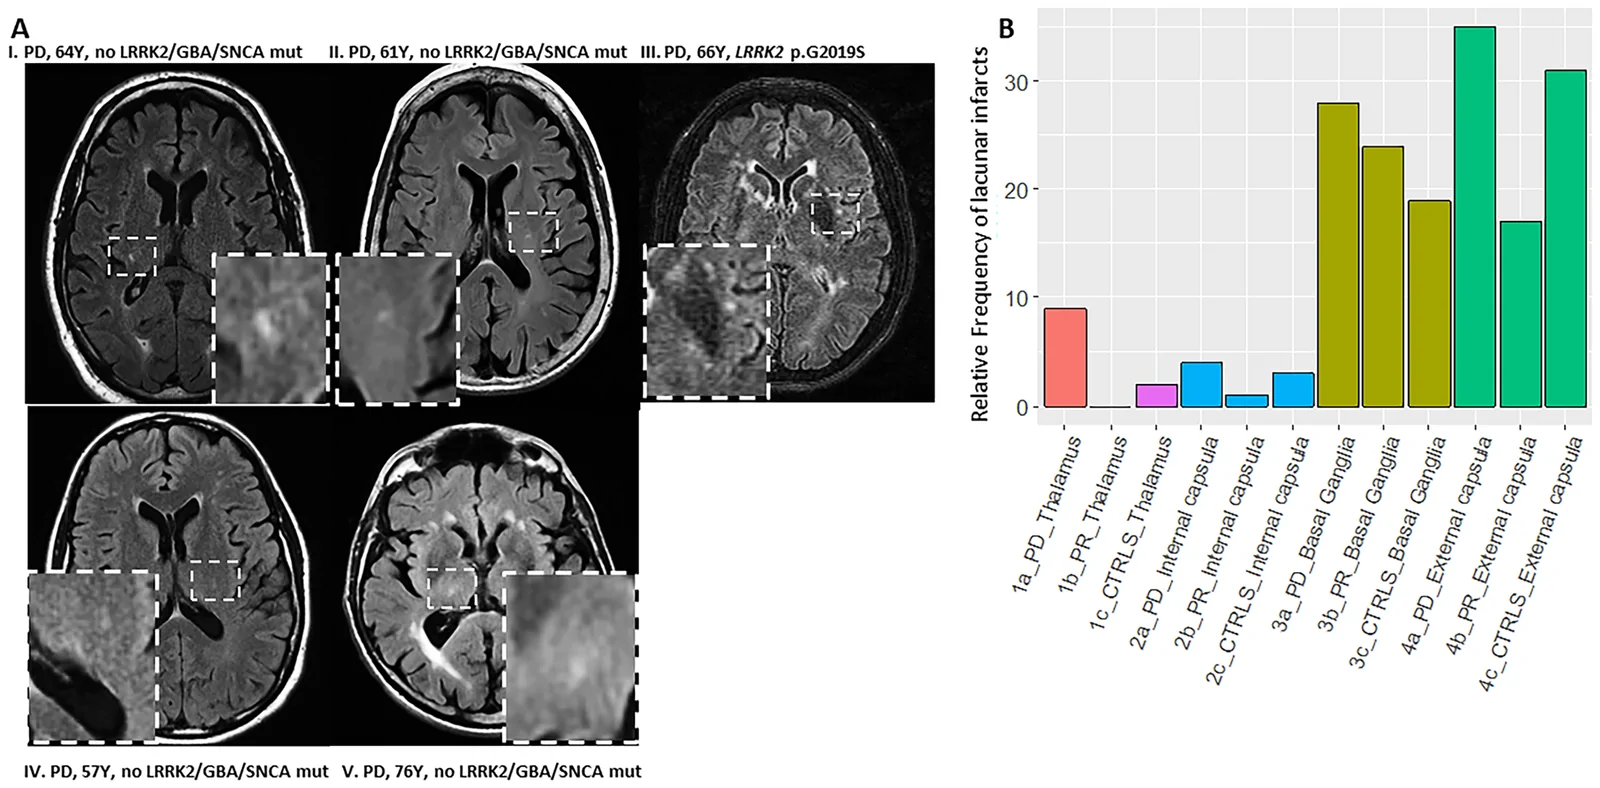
A I-V Axial T2-FLAIR MRI scans showing lacunar infarcts detected in 5 late-onset familial PD patients, framed within a dashed line. I Right thalamus infarct in a PD patient, 64 years old, not carrying any rare known pathogenic mutation in *LRRK2*, *GBA* and *SNCA*, heterozygous for *APOE* ε3 (ε2/ ε3), presenting a mild motor impairment (MDS-UPDRS-III 13) and mild cognitive impairment (MoCA 24). II, Left corona radiata infarct in a PD patient, 61 years old, not carrying any known rare pathogenic mutation in *LRRK2*, *GBA* and *SNCA*, heterozygous for *APOE* ε3 (ε2/ ε3), presenting a mild motor impairment (MDS-UPDRS-III 17) and no signs of dementia (MoCA 27). III Left capsula externa infarct in a PD patient, 66 years old, carrying *LRRK2* p.G2019S, homozygous for *APOE* ε3, presenting a severe motor impairment (MDS-UPDRS-III 48) and no cognitive impairment (MoCA 28). IV, Left thalamus infarct in a PD patient, 57 years old, not carrying any rare known pathogenic mutation in *LRRK2*, *GBA* and *SNCA*, homozygous for *APOE* ε3, presenting a severe motor impairment (MDS-UPDRS-III 31) and no cognitive deficits (MoCA 27). V, Right thalamus infarct in a PD patient, 76 years old, not carrying any rare known pathogenic mutation in *LRRK2*, *GBA* and *SNCA*, heterozygous for *APOE* ε4 (ε3/ ε4), presenting a mild motor impairment (MDS-UPDRS-III 18) and no cognitive deficits (MoCA 30) B. Relative frequency of different lacunar infarcts (thalamus, basal ganglia, capsula interna and capsula externa) detected in our cohort. PD, familial Parkinson’s disease patients; PR, familial prodromal Parkinson’s disease patients; CTRLS, controls.
